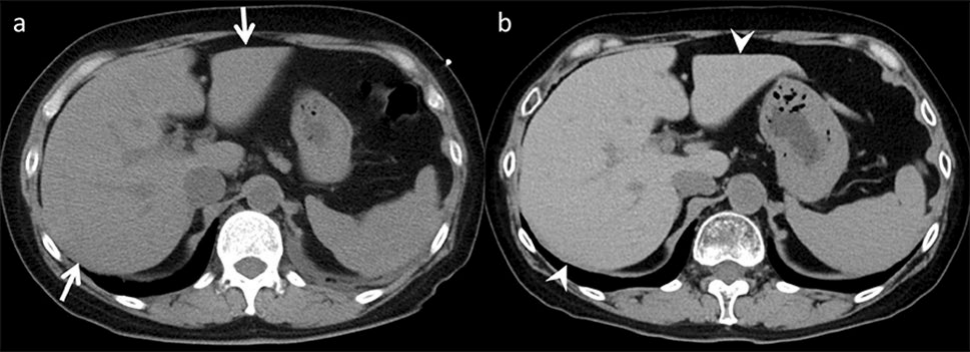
Secondary iron overload in a 61-year-old woman with malignant lymphoma, who was undergoing repeated blood transfusions for chemotherapy-induced myelosuppression. a Unenhanced computed tomography (CT) demonstrates normal liver attenuation (CT value, 46 HU) before the blood transfusions (arrows). b An increase in liver attenuation (75 HU) is observed on unenhanced CT performed 1.5 years after initiating blood transfusions (arrowheads). No hematological data or symptoms indicated abnormal liver function or hepatitis. It is difficult to differentiate iron overload from amiodarone deposition on a single image alone
